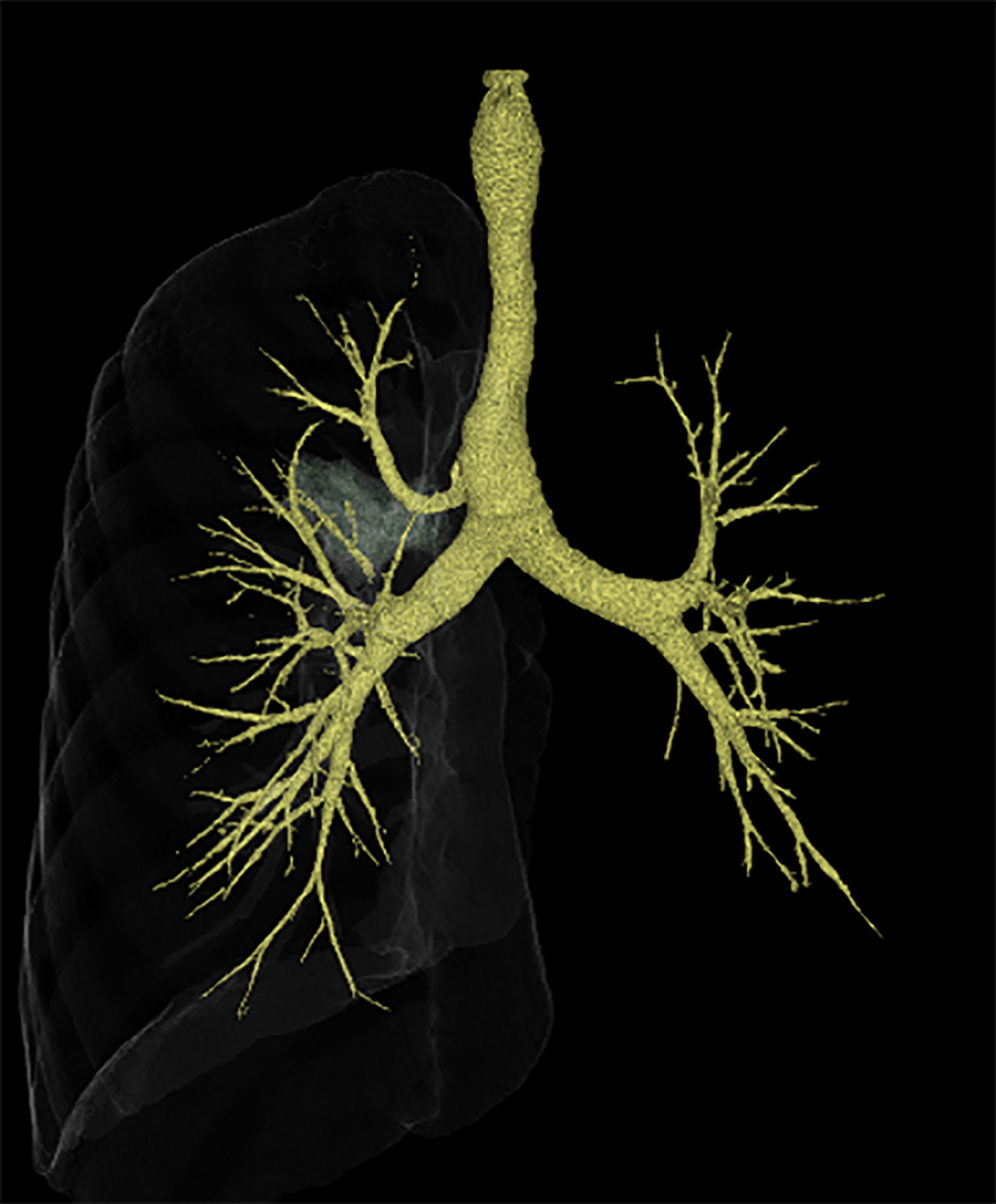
Computed tomography revealed a tracheal bronchus and a tracheal stenosis.
